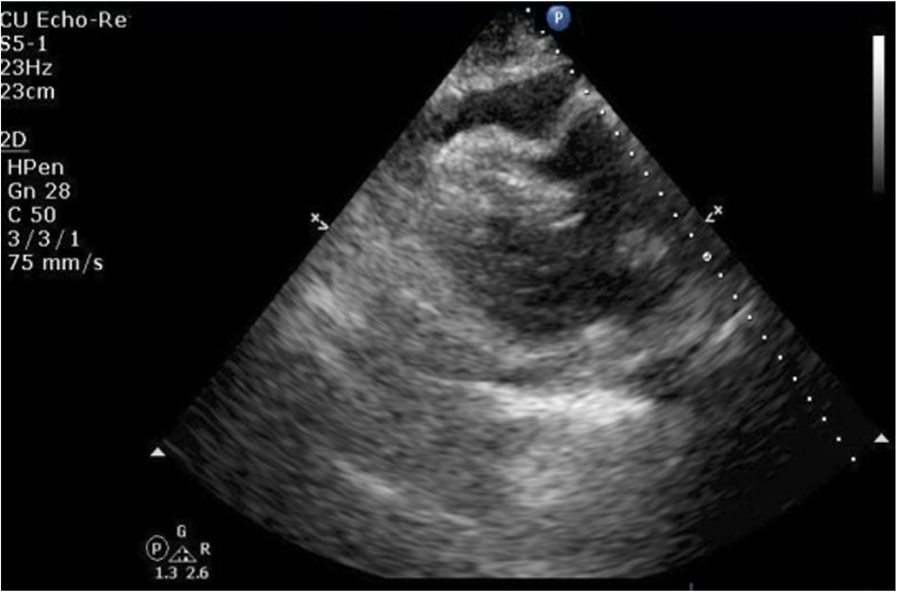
A transthoracic echocardiography showing the heart surrounded by moderate pericardial effusion seen as echo-free space more than 12mm with evidence of right ventricular collapse. Parasternal long-axis view with right ventricular collapse with fluid width about 1.44cm.
